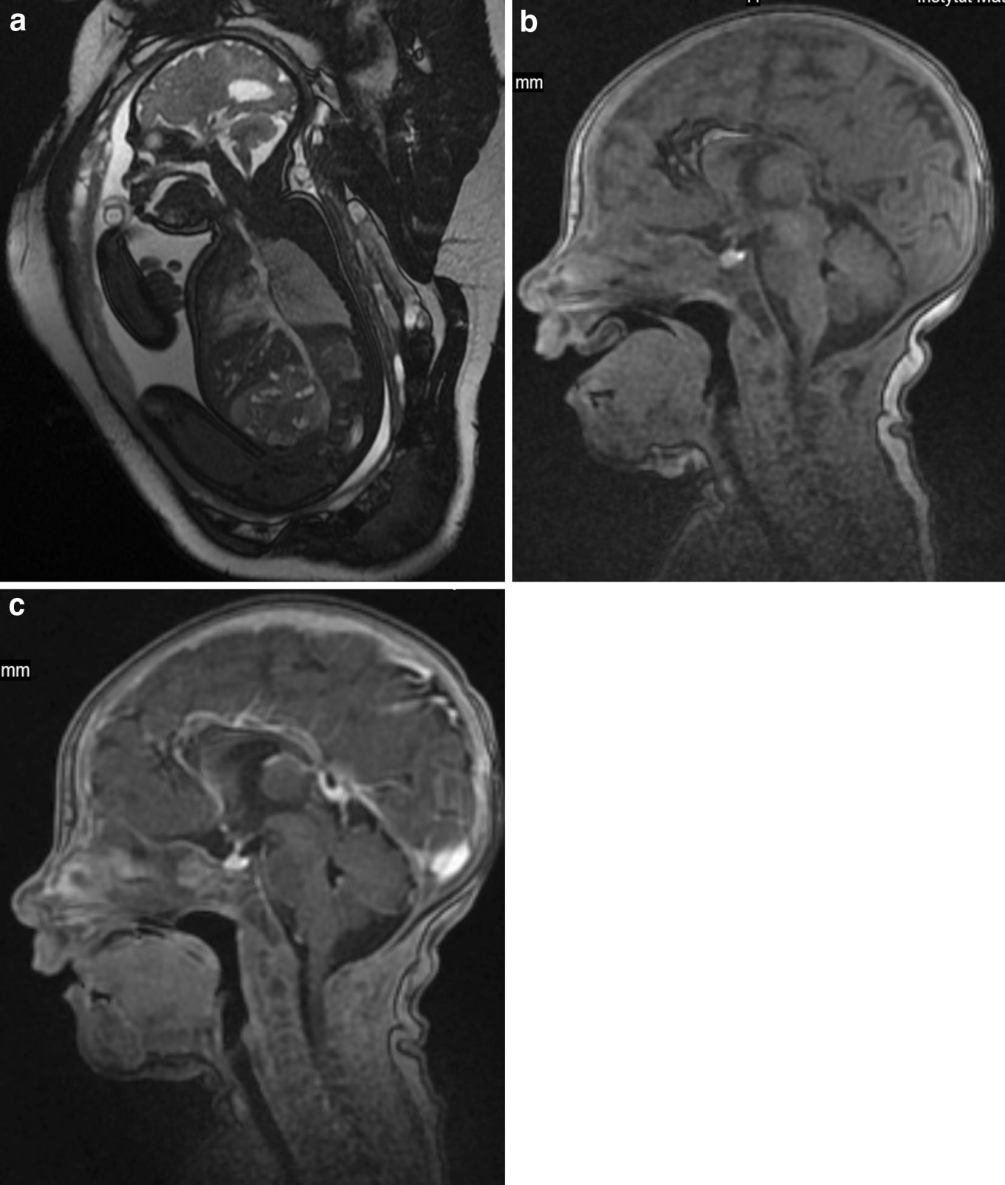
Case no. 22. a Fetal MRI. FIESTA/2D image, sagittal plane. The irregular contour of the palate is visible but impossible to assess as a tumor. b Postnatal MRI. 3D/T1-weighted image. The pedunculated nodule arising from the anterior part of the palate is well seen in this midsagittal section with the open mouth of the newborn. c Postnatal MRI. 3D/T1-weighted image after gadolinium injection, sagittal plane. With the mouth closed the nodule is no longer visible. One can only state that it does not enhance
